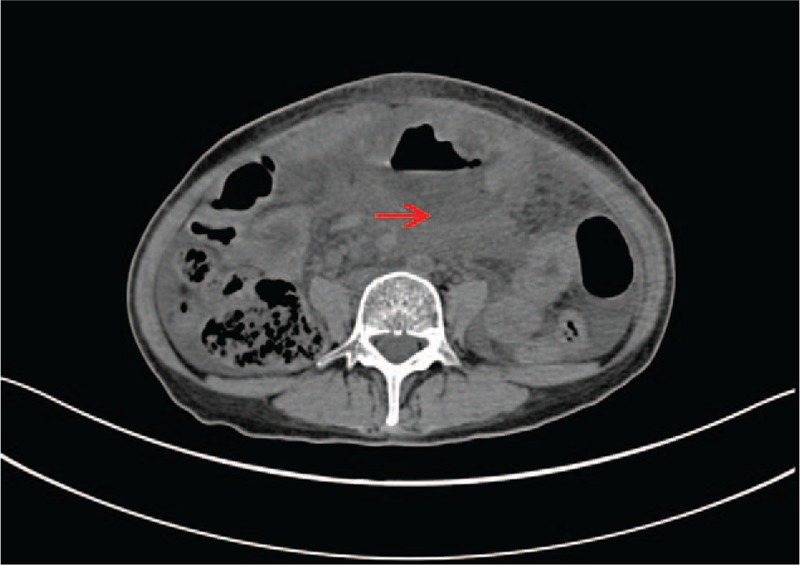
Abdominal CT scan 12 months after TP. It showed encapsulated effusion and gases surrounding the gastrointestinal anastomosis (red arrows).
